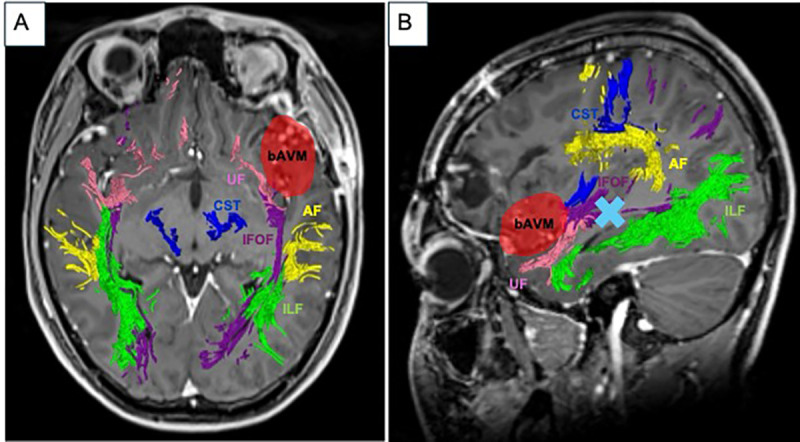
Representative post-contrast T1-weighted MRI brain images fused with post-processed DTI tractography in relation to the bAVM region (red) using Modus Plan (Synaptive Medical, Toronto, Canada) in (A) axial and (B) sagittal directions. The arcuate fasiculus (AF) is noted to be posterior to the bAVM. Of note, the light blue arrow in (B) depicts the region whereby direct cortical stimulation was applied and the patient demonstrated speech apraxia. (Abbreviations: AF = arcuate fasiculus, bAVM = brain arteriovenous malformation, CST = corticospinal tract, IFOF = inferior fronto-occipital fasiculus, ILF = inferior longitudinal fasiculus, UF = uncinate fasiculus).
